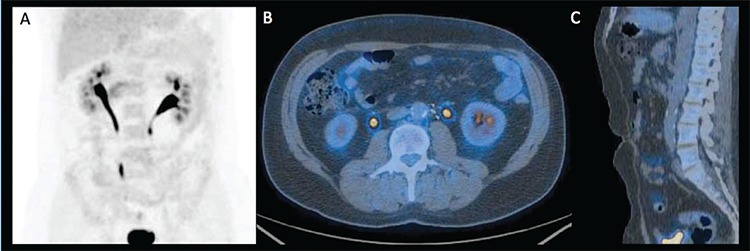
(A) MIP, (B) transaxial and (C) sagittal 18F-FDG-PET/CT fused image performed after 6 months from the beginning of therapy withprednisone and methotrexate which didn’t show 18F-FDG uptake.
